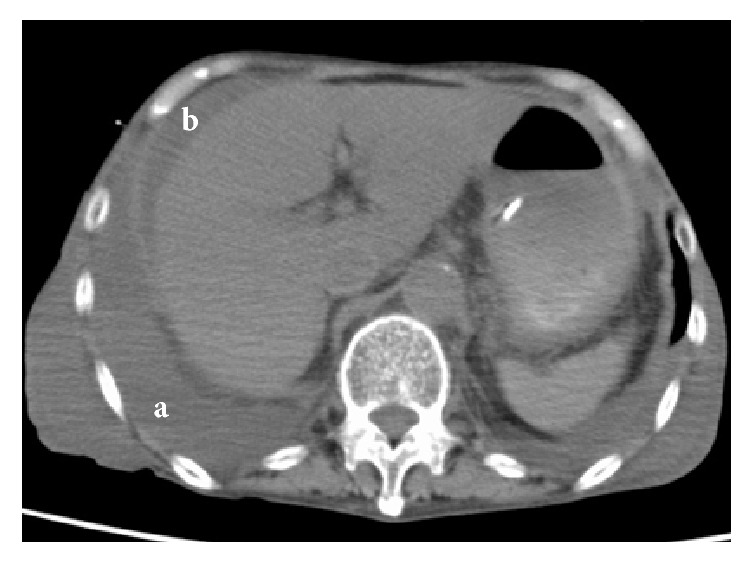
Computed tomography of the abdomen. Pleural effusion (a) and ascites (b) are seen. Within the liver, no abscess lesions were noted.
